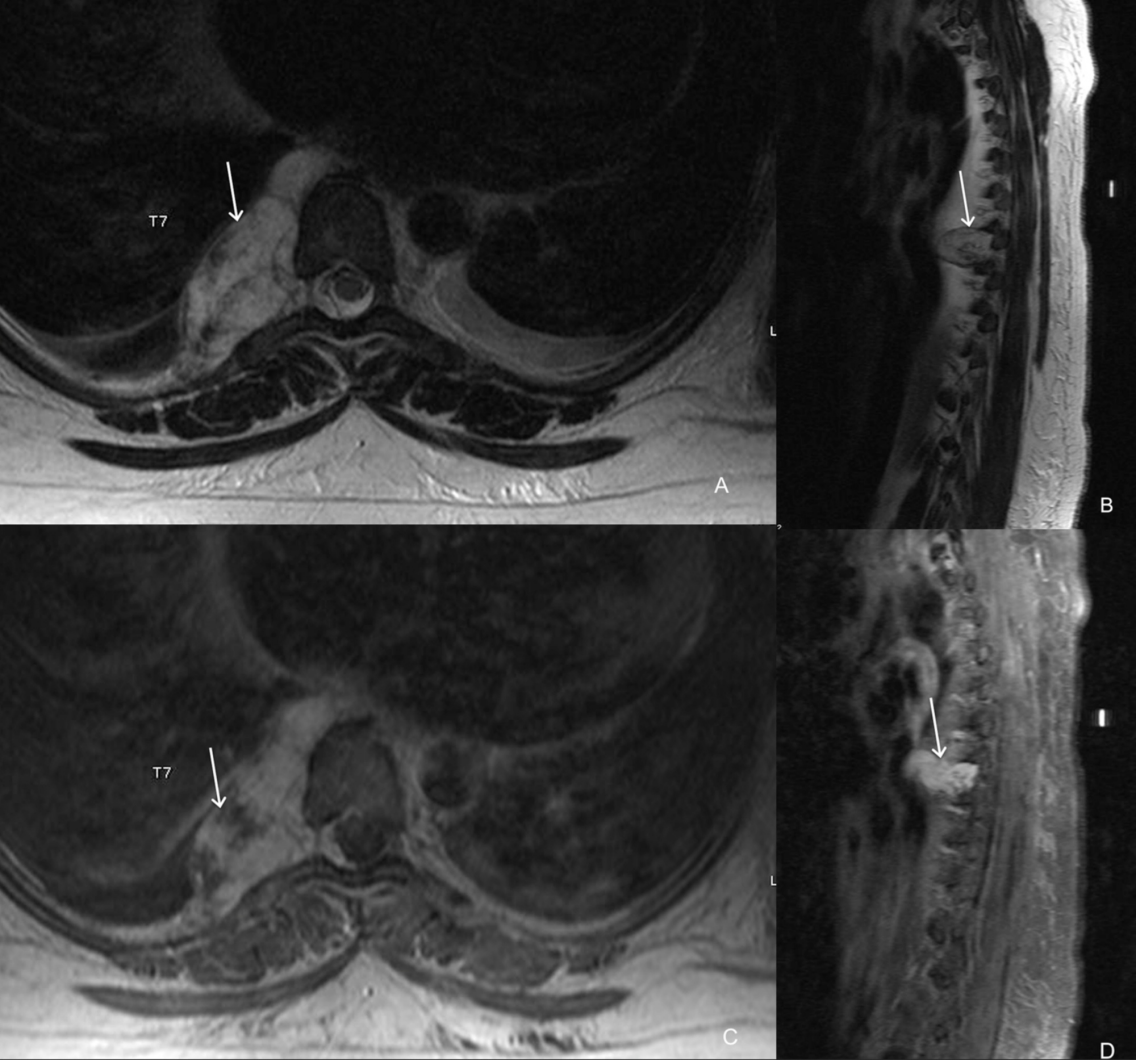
MRI of the thoracic spine, T2-weighted axial (A), sagittal (B) and contrast-enhanced T1-weighted axial (C) and sagittal (D) views demonstrate an avidly enhancing mass (arrow) extending through the right neural foramen and into the right lateral epidural space mildly indenting the right lateral thecal sac. The central canal and left neural foramina are widely patent.
